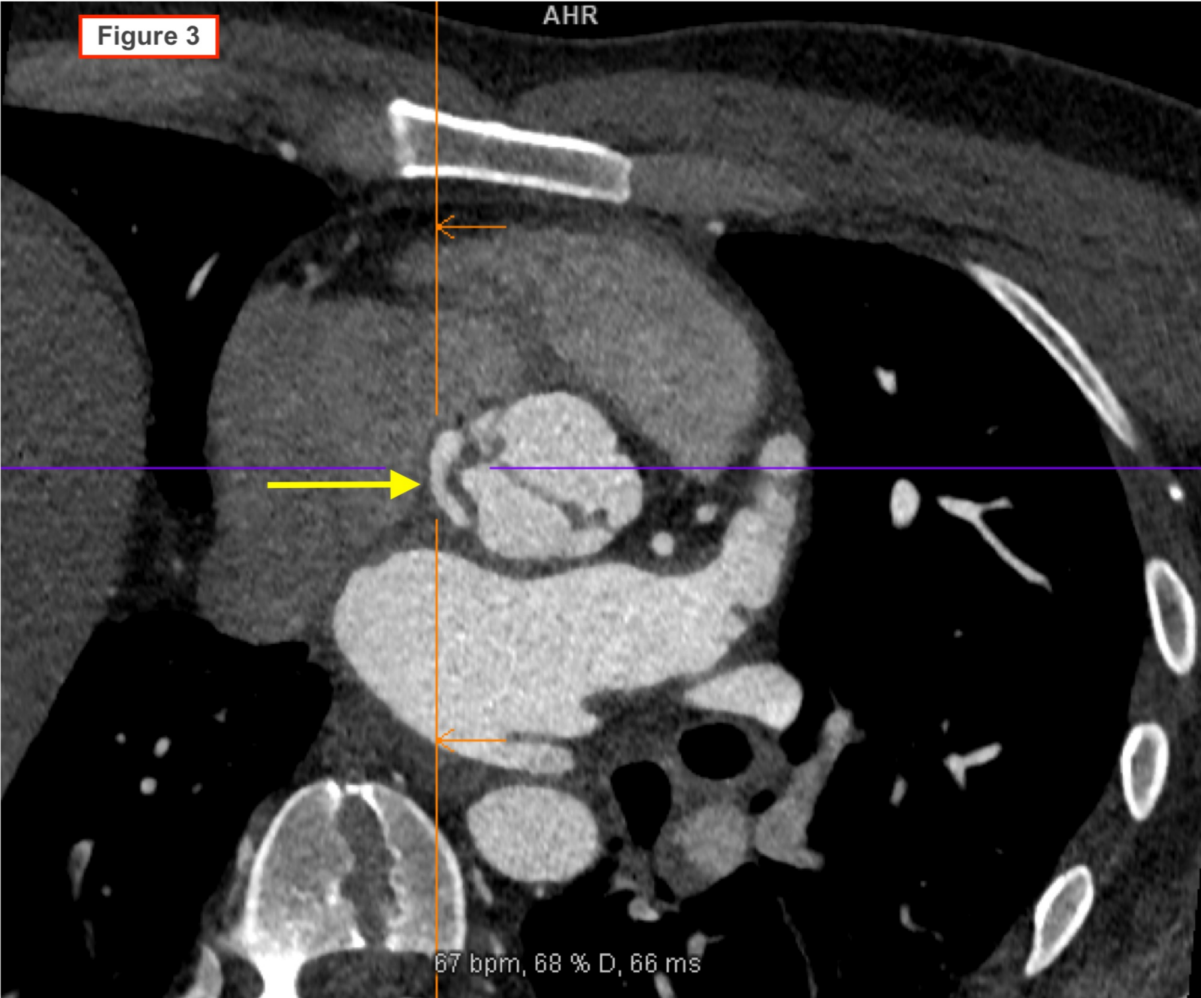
Cardiac CT image showing a fistula from the base of the left ventricle into the left atrium.
